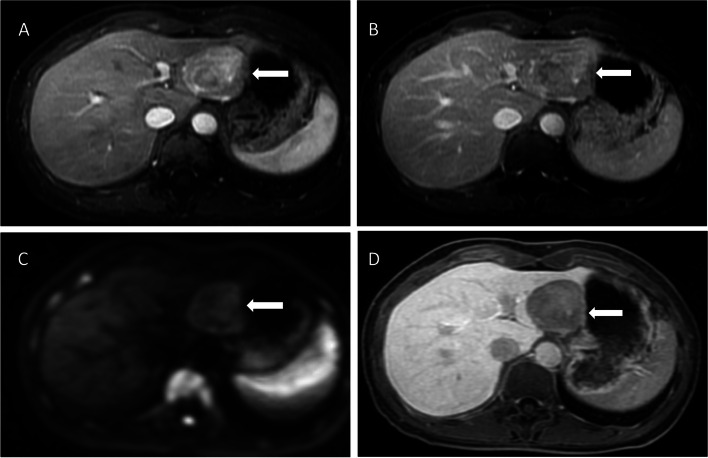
A and B Contrast-enhanced T1-weighted MR images with hepatobiliary contrast agent show arterial hyperenhancing mass in left hepatic lobe (arrow in A) with wash-out appearance on delayed phase (arrow in B). C Diffusion weighted MR image with high B value shows hyperintense mass compared to the surrounding hepatic parenchyma. D Hepatobiliary phase MR image shows hypointense mass compared to the surrounding hepatic parenchyma
